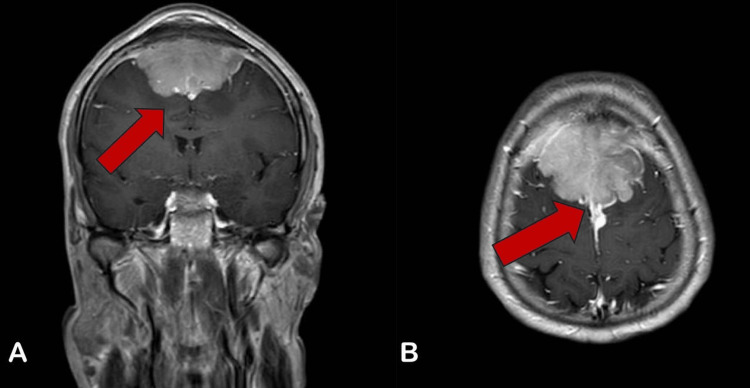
(A) Coronal T2-Weighted MRI Demonstrates the Mass Effect on the Anteromedial Portion of Bilateral Frontal Parenchyma. (B) Axial T2-Weighted MRI Shows Complete Invasion to the Middle Third of the Superior Sagittal Sinus.
